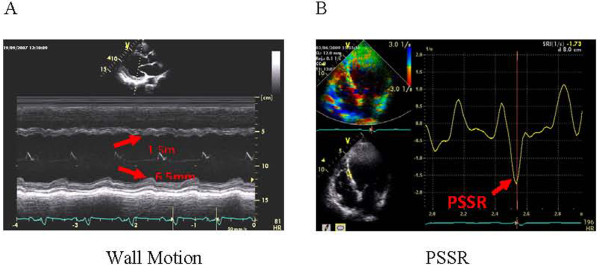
Imaging methods. ( A) Amplitude of regional myocardial motion was the range of motion measured using M-type echocardiography. ( B) PSSR was the measurement of the peak systolic strain rate using tissue Doppler imaging.
